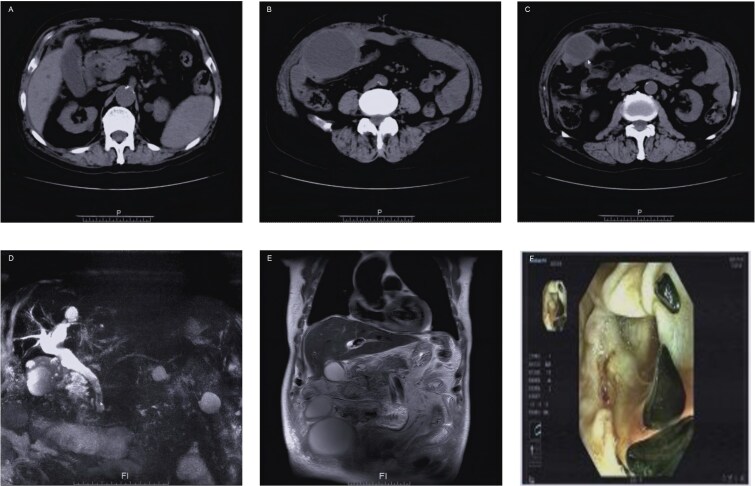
(A, B, C) Plain abdominal CT scan; (D, E) pancreatic bile duct hydrography MRCP; (F) endoscopic retrograde cholangiopancreatography.
